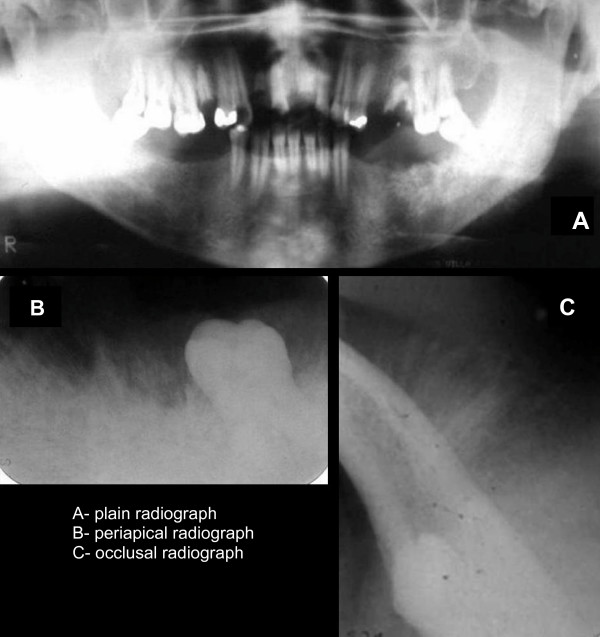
Orthopantomography (A) and occlusal (B) and periapical (C) radiographs reveal diffuse bone destruction on the left side of the mandible, presenting dense radiopaque, mixed and completely radiolucent areas. The periosteum showed the classical "sunray" reaction on occlusal (B) and periapical radiographs (C).
